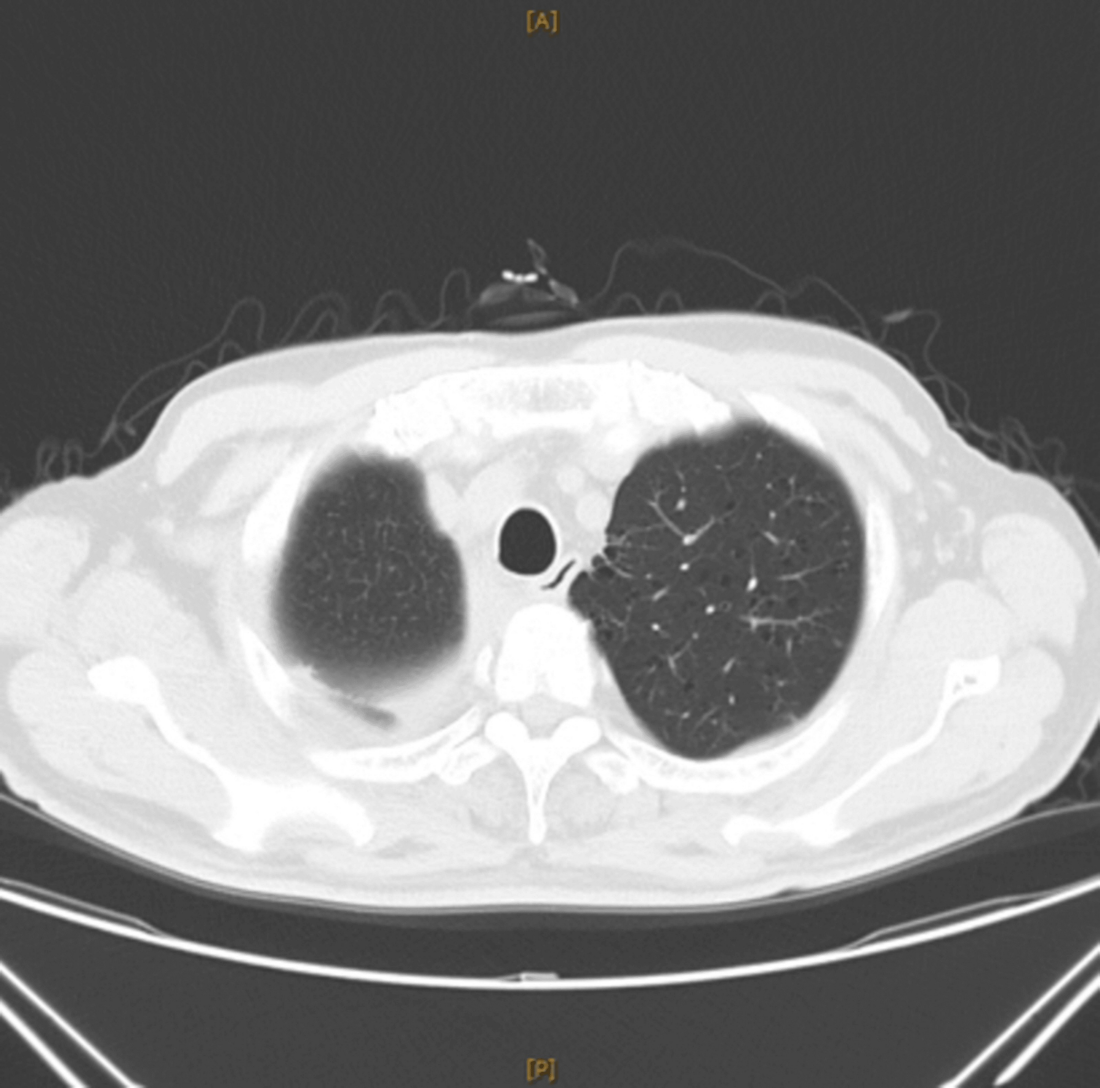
CT after resection of lung cancer showing no significant mass is observed in the right upper lung after tumor resection
